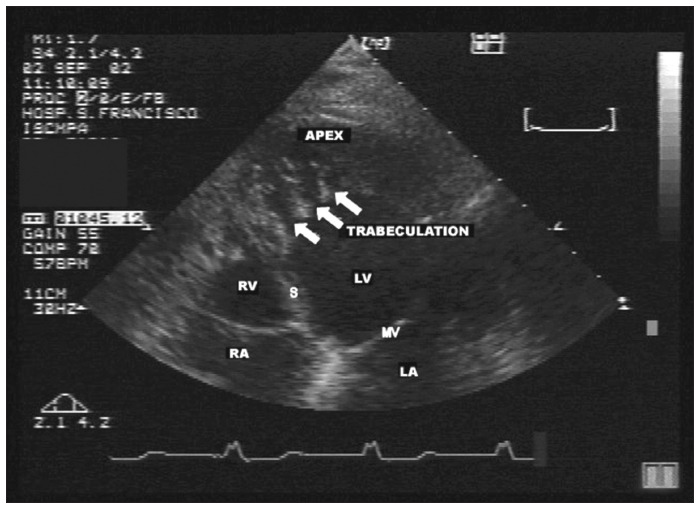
Four-chamber image of the same patient as in Figure 1, showing prominent apical left ventricular trabeculation (white arrow), for whom a diagnosis of noncompaction was suggested. The patient had previously been given an echocardiographic diagnosis of endomyocardial fibrosis.
